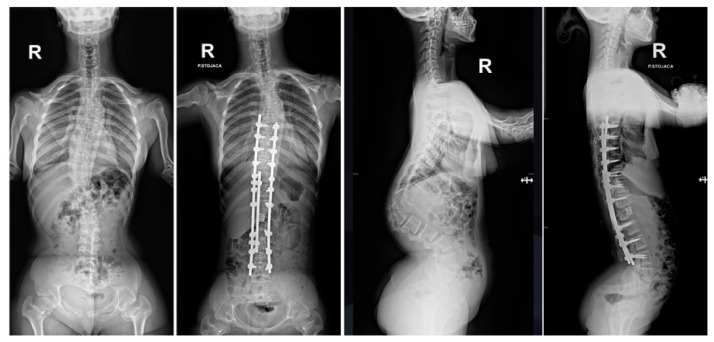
Postoperative X-rays after surgical treatment in comparison with pre-operative X-rays.
